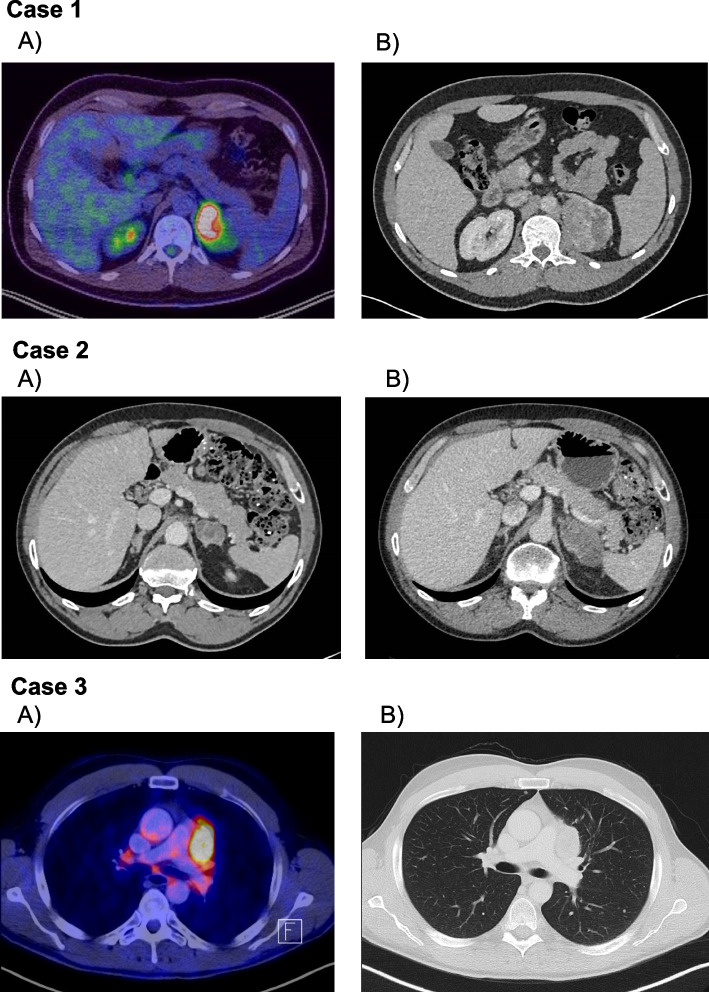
Radiological imaging of large adrenocortical lesions before surgery or at the time of disease recurrence. Case 1 (at time of diagnosis). Panel a) FDG-PET CT scan December 2019: The left adrenal mass is concordant with the CT scan from November. The lesion shows abnormal FDG uptake with maximum S U V is 16.2. The lesion remains highly suspicious of soft tissue metastasis from melanoma. Panel b Abdomen Pelvis CT scan April 2020: The left adrenal mass measures up to 73 mm maximum axial dimension. It is closely related to the medial aspect of the spleen where direct invasion cannot be excluded, to the left crus of the left kidney but there are thought to be thin planes of separation from these structures. No evidence of other metastatic disease.of the diaphragm and to the upper pole. Case 2 (at time of diagnosis). Panel a and Panel b) Thorax Abdomen Pelvis CT scan October 2020 – Panel b (compared to July 2020 – Panel a): Further enlargement of the isolated multicystic left adrenal metastasis, now 62.0 mm × 32.5 mm (compared to 33 × 26 mm in 3 months’ interval). The metastasis now abuts the stomach and spleen as well as the pancreas. Multi-planar reconstruction suggests the stomach rugae are lying against the metastasis rather than being invaded by it. No evidence of other metastatic disease. C) Case 3 (at time of disease recurrence). Panel a) FDG-PET CT scan and b) Abdomen Pelvis CT scan March 2015 at time of tumor progression. Evidence of lung metastases and new lymph nodes metastasis in the mediastinum
